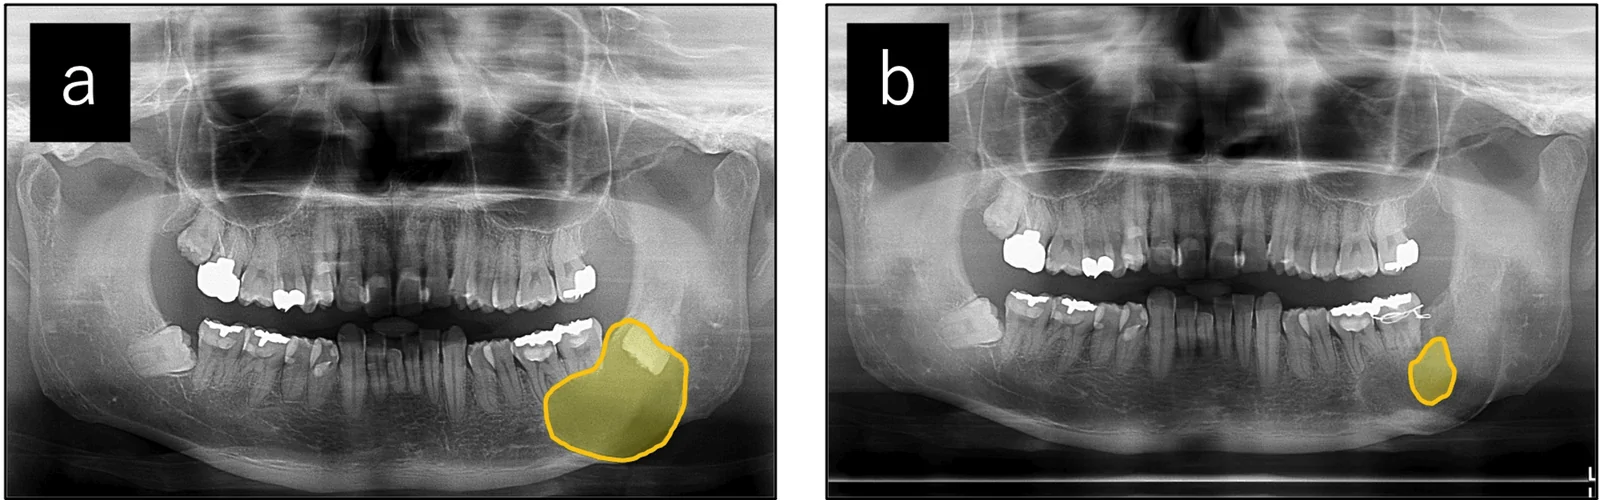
Measurement of lesion reduction rate using radiographic imagery. (a) Pre-decompression panoramic radiograph. (b) Post-decompression panoramic radiograph. The orange/yellow outlined and shaded areas represent the pre- and post-decompression lesion areas, respectively, which were segmented for pixel-based area quantification. Source: Patient radiographs obtained from the authors' clinical repository.
